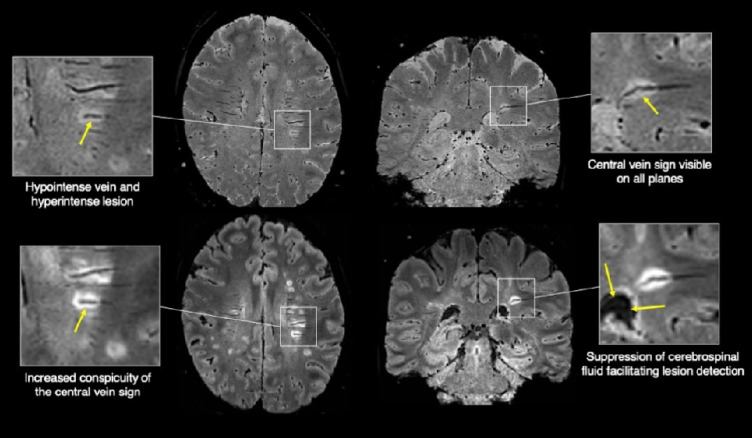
MRI technique for MS detection.
